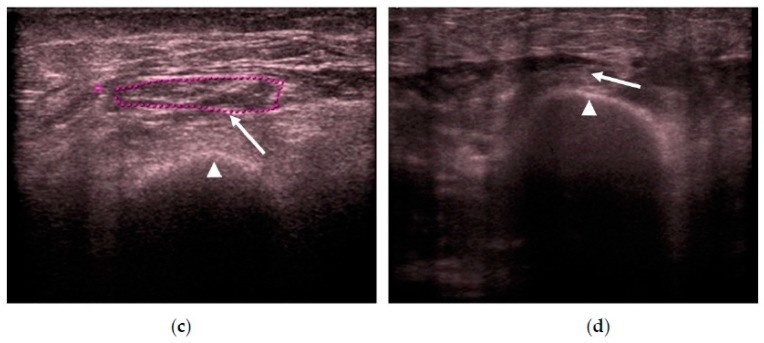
Transversal ultrasonography (US) scan that shows quadriceps rectus femoris (QRF) muscle echogenicity. (a) Matched healthy control: Category 1 (normal hypoechogenicity), with the central tendon (thick arrow). (b) Category 2 (heterogeneous). (c) Category 3 (fat infiltration). (d) Category 4 (atrophy due to fasciitis and muscle necrosis). QRF: quadriceps rectus femoris (arrows); femur (arrowhead); ST: subcutaneous cellular tissue (dotted line); US: ultrasound.
